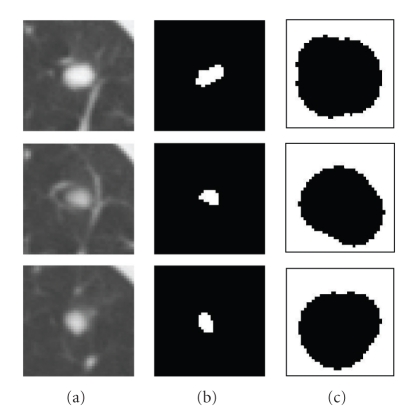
An example of the initialization based on spherical concentration on an attached nodule. (a) 3D nodule in three contiguous CT slices; (b) initial foreground based on high spherical concentration; (c) initial background region.
